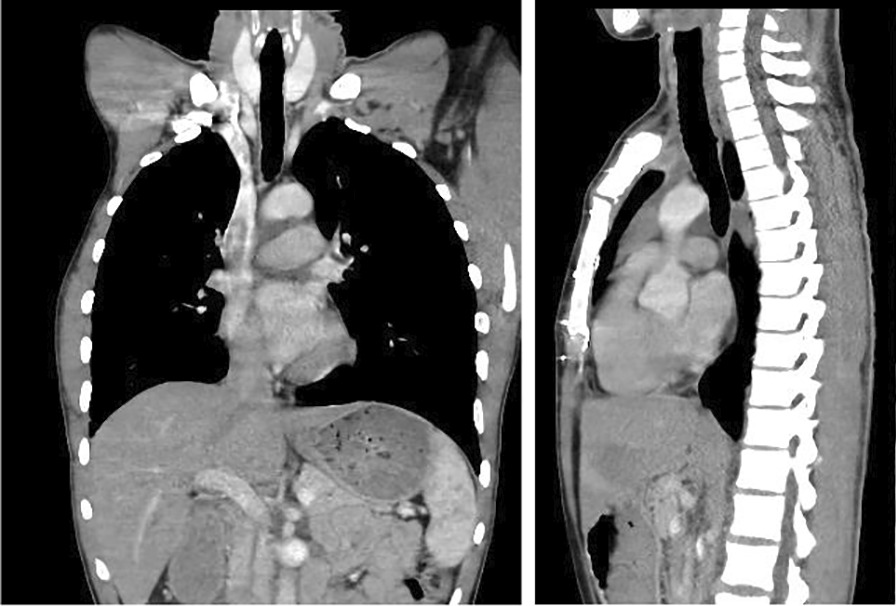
Unremarkable Chest computed tomography scan done after two years following tracheal resection
